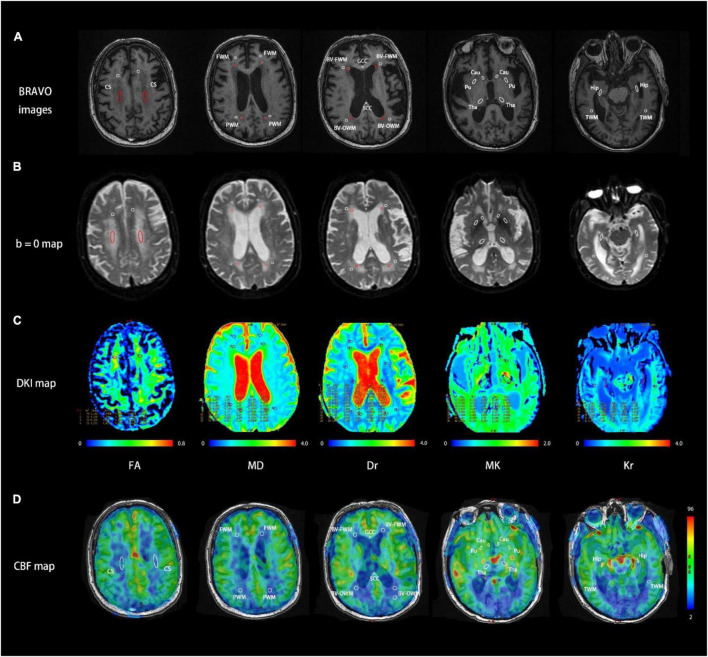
Representative MR images at five consecutive slices of a patient with Binswanger’s disease (BD) acquired using BRAVO image (A), DKI *b* = 0 map (B), DKI parametric maps (C), and cerebral blood flow (CBF) map (D). Region of interest (ROI) settings. CS, centrum semiovale; GCC/SCC, genu/splenium of the corpus callosum; FWM/PWM/TWM, frontal/parietal/temporal white matter; BV-FWM/BV-OWM, frontal/occipital white matter around lateral ventricle; Cau, caudate nucleus; Pu, putamen; Tha, thalamus; Hip, hippocampus. Each ROI was also denoted with BRAVO images, *b* = 0 maps, and DKI/ASL-derived parameters maps. The red ROIs in *b* = 0 maps and BRAVO images represent white matter hyperintensities and the white ROIs in both maps represent normal areas in MRI.
